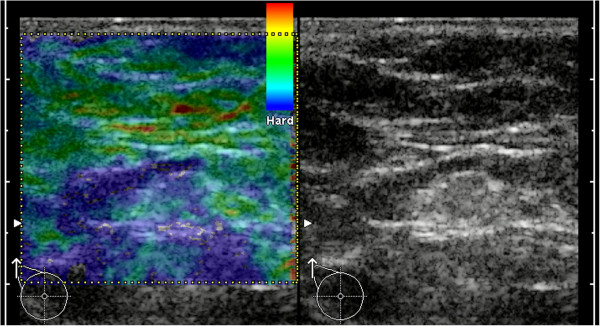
Example for B-mode ultrasound and elastogram of a metastatic LN. In B-mode ultrasound, the cortex of the LN is slightly enlarged (maximum ~3.5mm). The predominant color of the medulla is turquoise (to green) and the cortex is mainly blue. Applying the criterion of a blue cortex, this case would be a true-positive.
